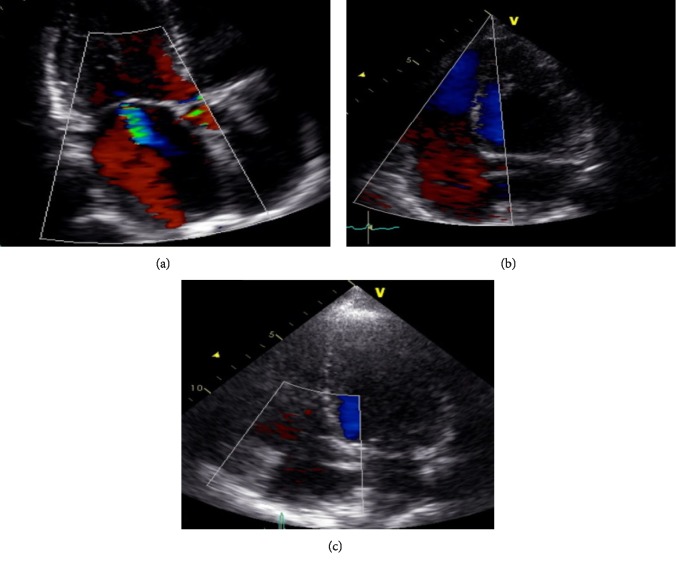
Echocardiography before (a), 2-days (b), and 3-months (c) after occlusion of the proximal high-flow renal arteriovenous fistula (RAVF) by Amplatzer Vascular Plug (AVP) Device II placement into the left renal artery (LRA). (a) 4-Chamber view with Doppler ultrasound found a functional (secondary) tricuspid regurgitation (Grade I). Recognize the enlargement of the right atrium due to increased volume load. (b and c) 4-Chamber view with Doppler ultrasound found no tricuspid regurgitation 2-days and 3 months after RAVF occlusion. Also recognize normalization of the right atrium dimensions due to reduced volume load.
